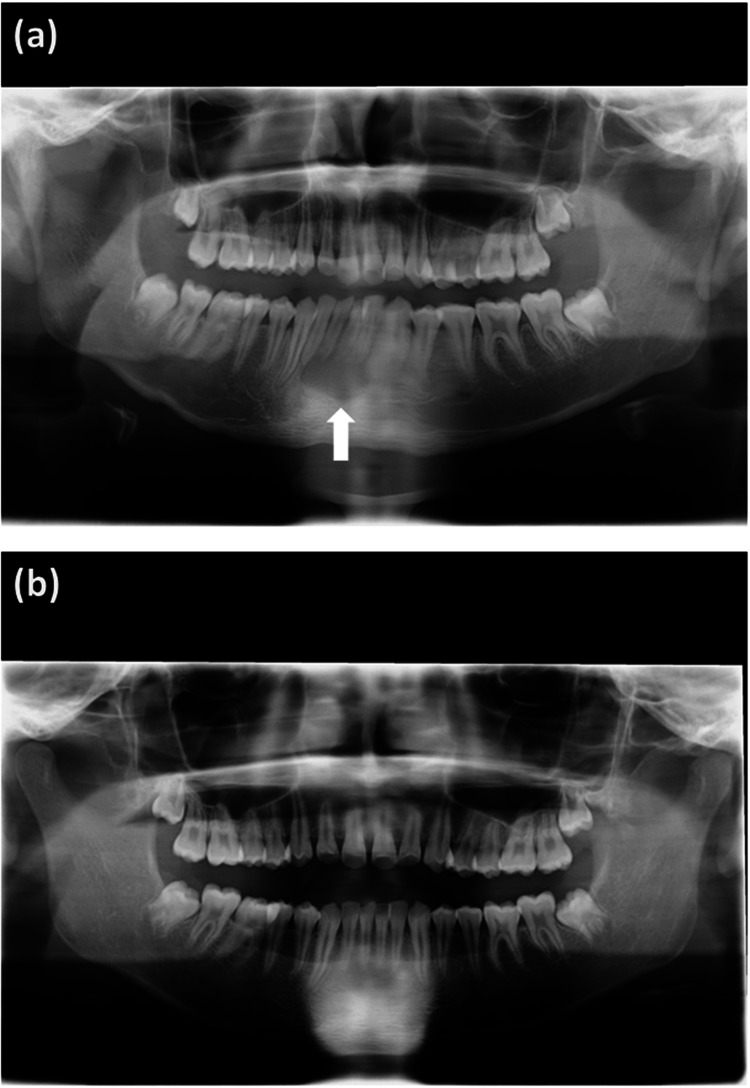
Panoramic radiographs of simple bone cyst in mandibular bone. (a) Day 3 of our treatment. (b) Eight months after our treatment.
